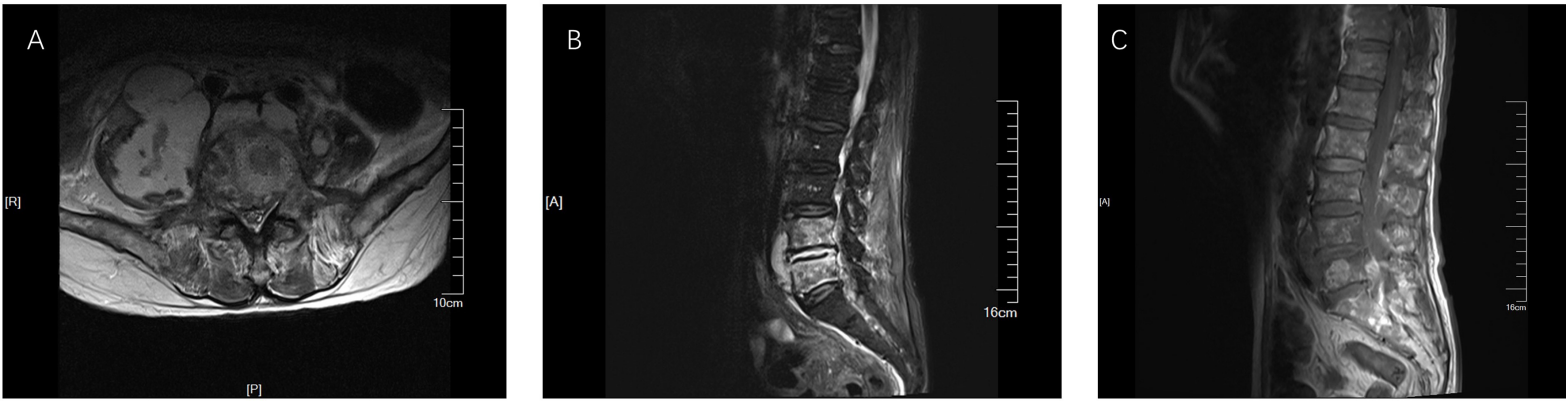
MRI results of lumbar plain scan. (A) The result of TIWI; (B) The result of T2FS; (C) The result of T2WI. The right lumbar vertebrae 4 and 5 showed high signal, and the signal intensity of L4-5 and L5-S1 increased. Abnormal signal was seen around lumbar 4-5 vertebral body, T1WI was equal signal, T2WI was slightly high signal, and lipid pressure image was high signal. The right psoas major, iliopsoas major, and left psoas major were swollen. Irregular abnormal signal shadow was seen in the course area, with uneven internal signal. The lesions on the right side were large, and the maximum axial plane was about 8.1 × 6.0cm.
